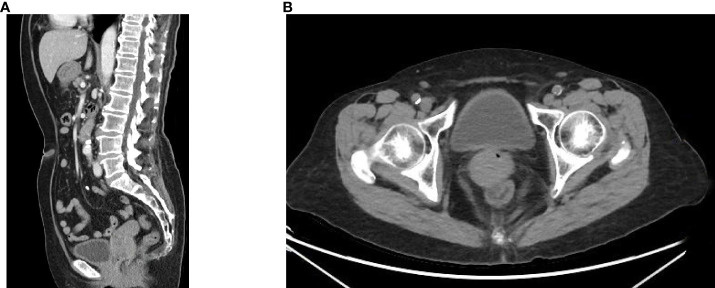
The CT scan before treatment revealed an enlarged cervix with inhomogeneous enhancement.
